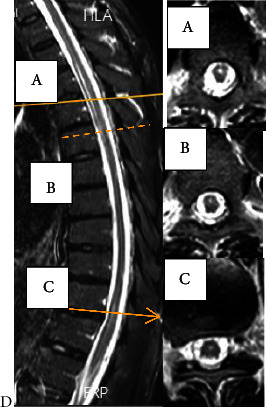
Thoracic MRI after 12 days of treatment. Lesion is better visible around T4-T6 (A and B) and T11-T12 (C).
